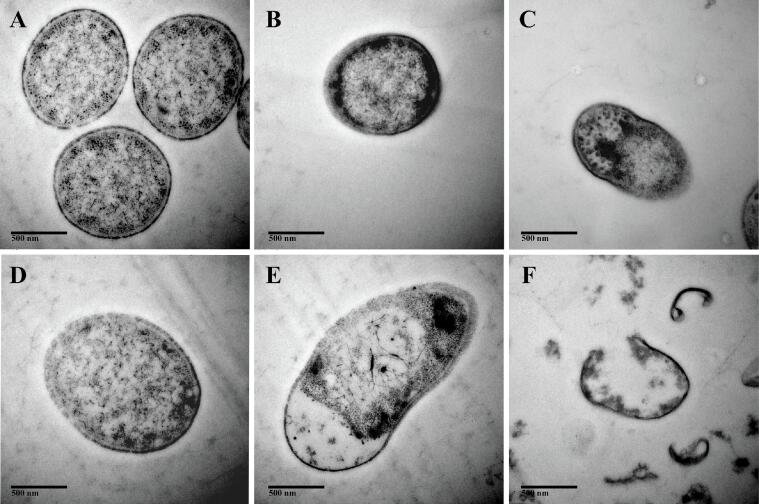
TEM-based observations (40,000 × magnification) of untreated *Salmonella* (A) and *Salmonella* treated with ultrasound (230 W/cm^2^) for 6 min (B), and *Salmonella* treated with ultrasound (345 W/cm^2^) for 9 min (C), and *Salmonella* treated with LEON at 0.08 μL/mL for 6 min (D), and *Salmonella* treated with LEON + US (230 W/cm^2^) for 6 min (E), and *Salmonella* treated with LEON + US (345 W/cm^2^) for 9 min (F).
